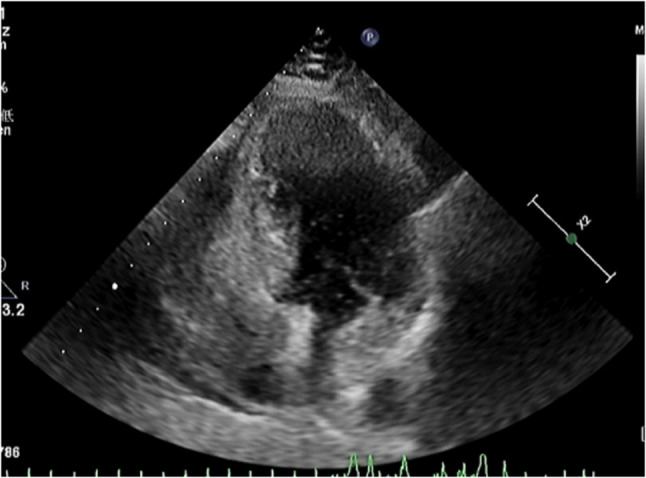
Routine preoperative right heart contrast echocardiography for the patient’s PFO closure procedure revealed a large number of microbubbles in the left heart
